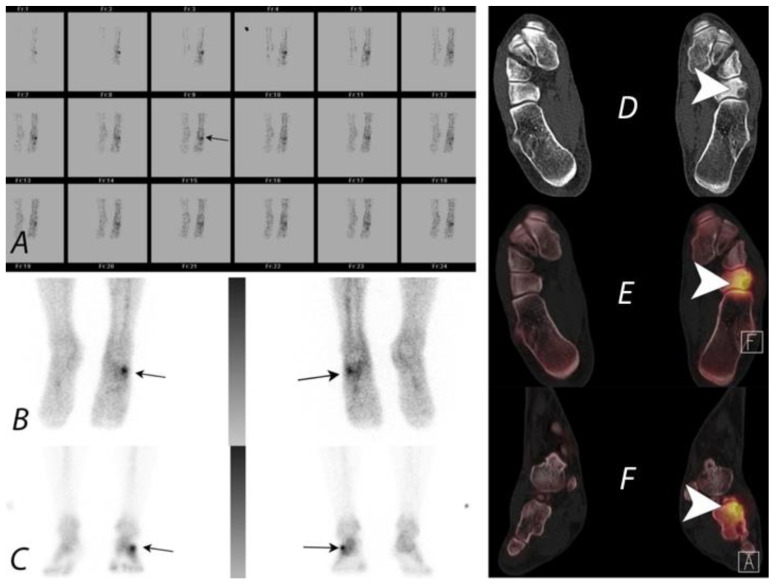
A young patient presenting with chronic left foot pain underwent three phase bone scan with SPECT/CT. Radiographs were interpreted as normal (not shown). Blood flow and pool images (A,B) demonstrate increased blood flow and pool activity with focal accumulation of activity on the delayed images (C) along the lateral aspect of the mid foot (black arrows). Axial CT image (D) and fused axial (E) and fused coronal (F) SPECT/CT images localize the activity to a well-defined lytic lesion with (white arrowheads) well defined sclerotic rim and a nidus involving the cuboid bone and was interpreted as a possible osteoid osteoma. Patient underwent biopsy, which revealed the lesion to be a benign cartilaginous neoplasm.
